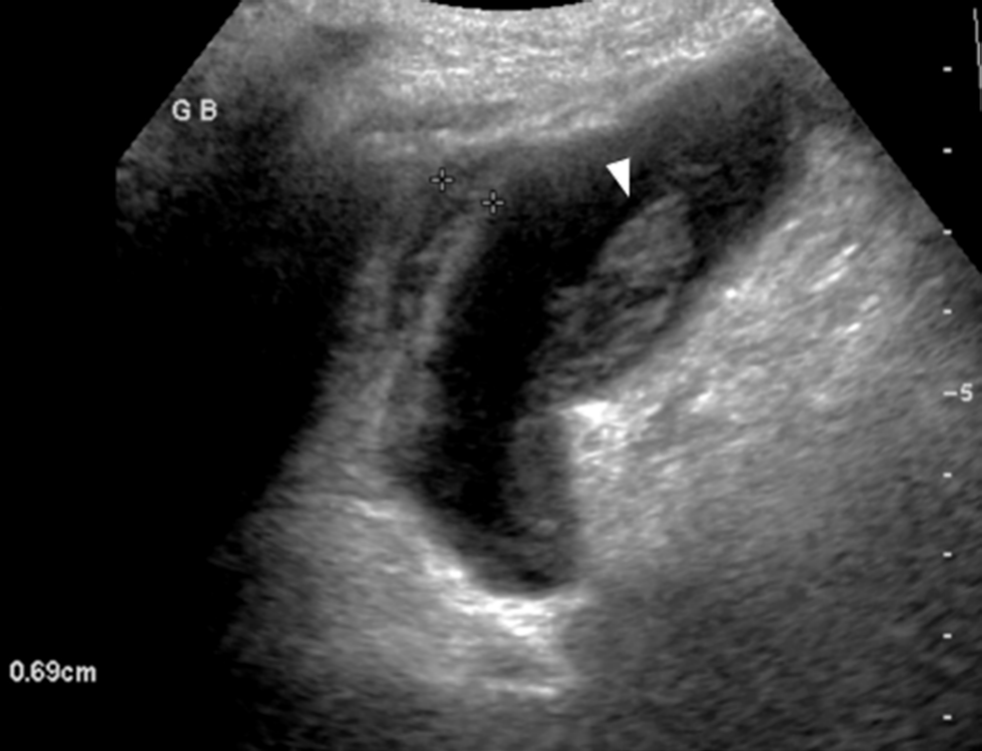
Acalculous acute cholecystitis in a critically ill 82-year-old female with altered mental status, increasing leukocytosis and right upper quadrant tenderness.Long axis ultrasound image of the gallbladder demonstrates thick and striated wall (between calipers), and echogenic sludge (arrowhead). No echogenic stone with posterior shadowing present
